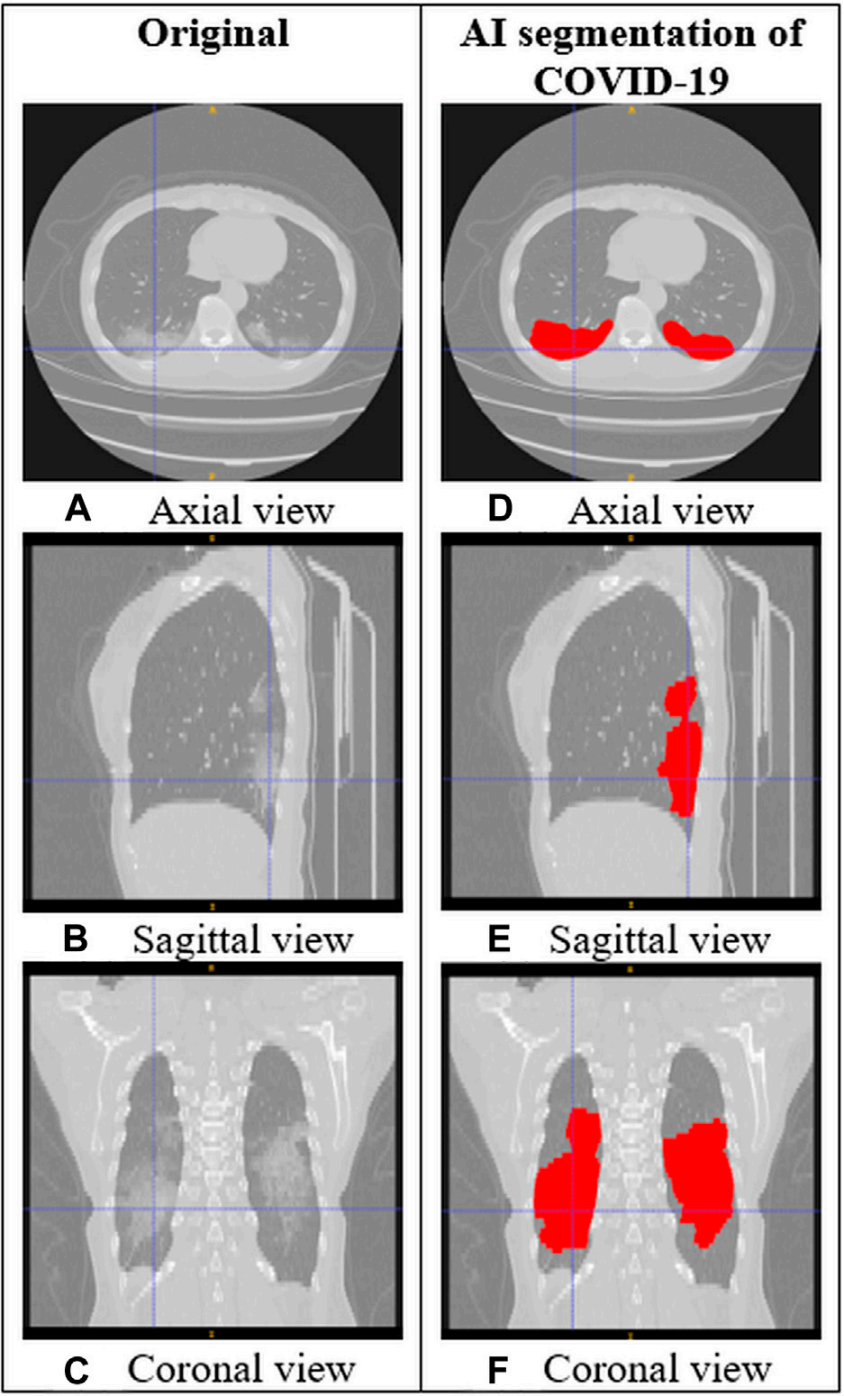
CT images of the lungs in a subject with COVID-19. (A–C) Original CT images. (D–F) CT images with AI segmentation of COVID-19 lung opacities.
